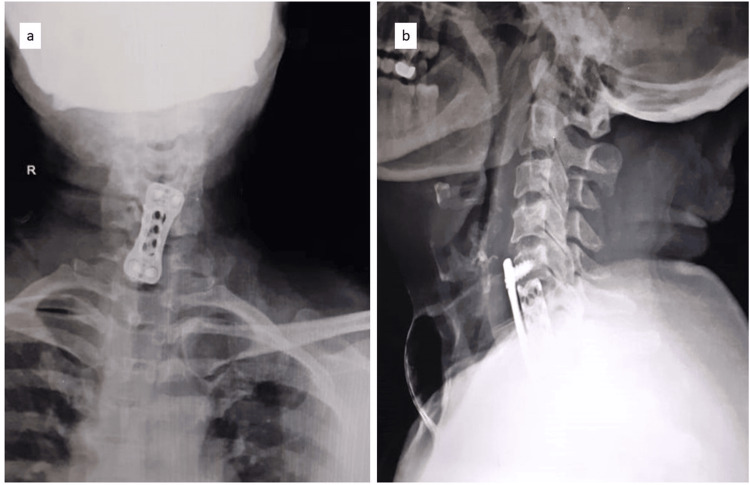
Postoperative radiographs after ACCF of the cervical spine. Postoperative radiographs showing C6 corpectomy with C5-C7 fusion, MESH cage, and anterior cervical plate; (a) anteroposterior radiograph, (b) lateral radiograph ACCF: anterior cervical corpectomy and fusion
